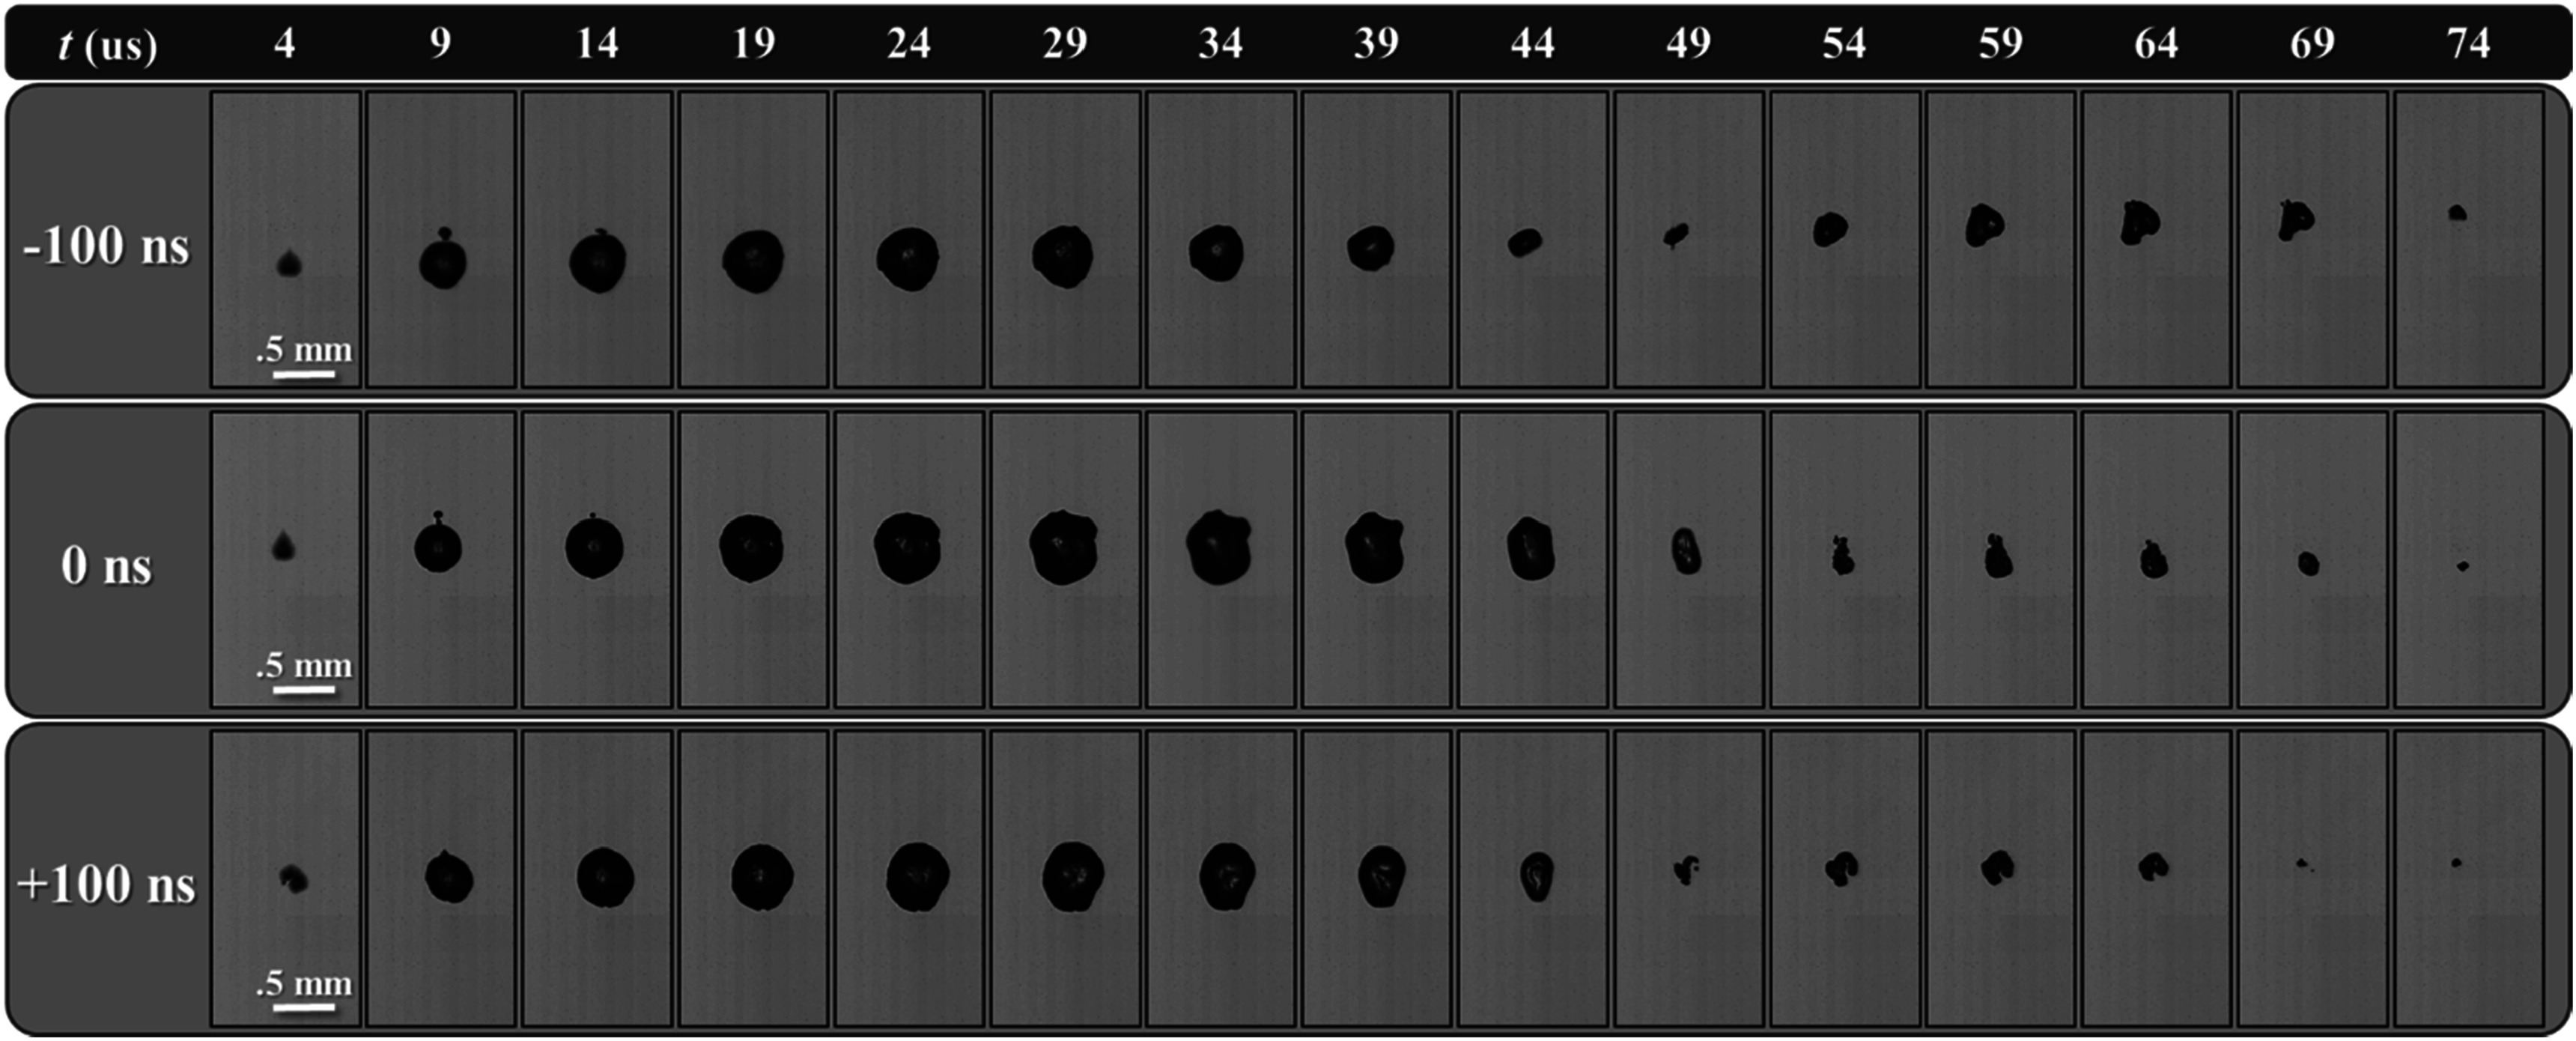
Optical single bubble size and dynamics comparison. Example optical images shown between 4–74 *μ*s after pulse arrival taken slightly above the respective cavitation thresholds for each 3 MHz arrival time captured by high-speed optical imaging at 5 *μ*s intervals. Ultrasound propagating from bottom to top.
